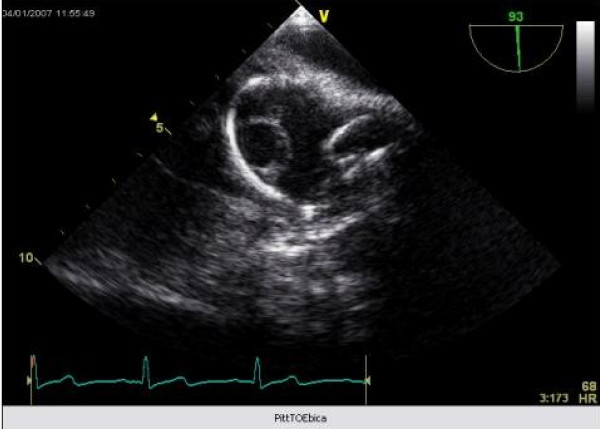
Transthoracic echocardiogram showing vegetations in relation to the endocardial pacing lead.
